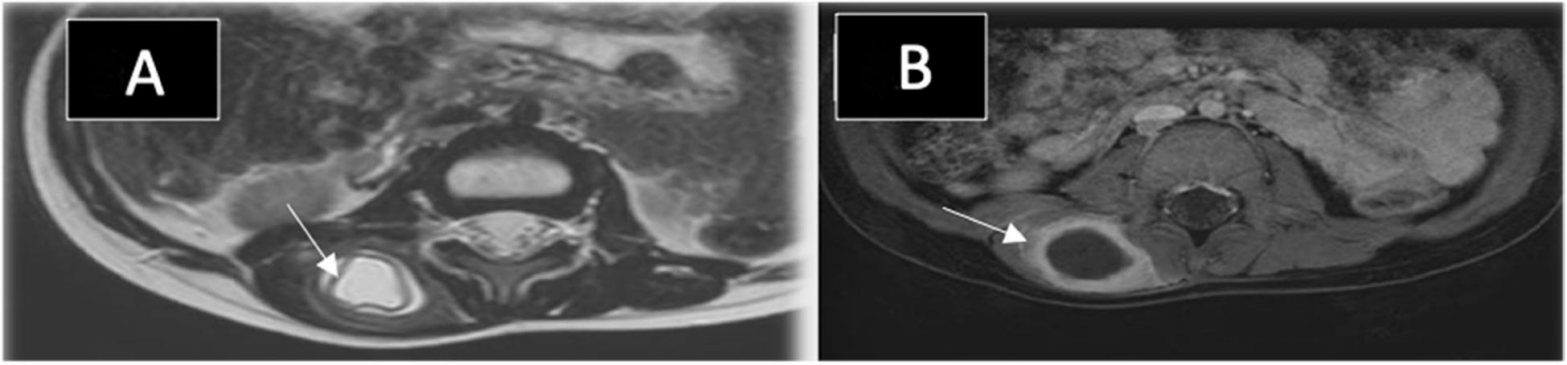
MRI of the lumbar spine in the axial planes. a: Axial T2 weighted images demonstrating the right Paraspinal hydatid cyst with water Lilly sign (arrow). b: Axial T1 postcontrast MRI with fat sat showing thick enhancement of the cyst capsule (arrow)
